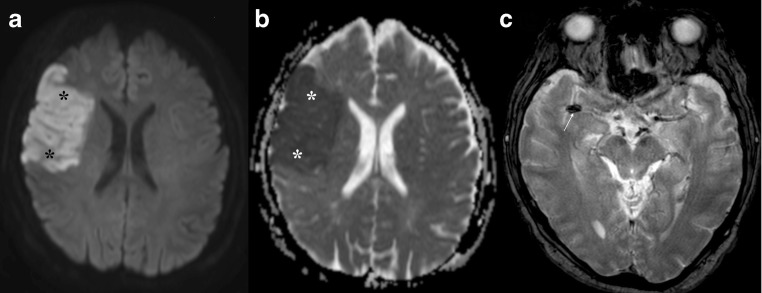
Acute stroke. a Increased signal intensity on DWI in the territory of the right middle cerebral artery (MCA), which corresponds to an area of low ADC value in b (asterisks), representing the extension of an acute ischaemic stroke. c A clot as the aetiology, presenting as a linear hypointense abnormality in the MCA on T2*WI (arrow)
